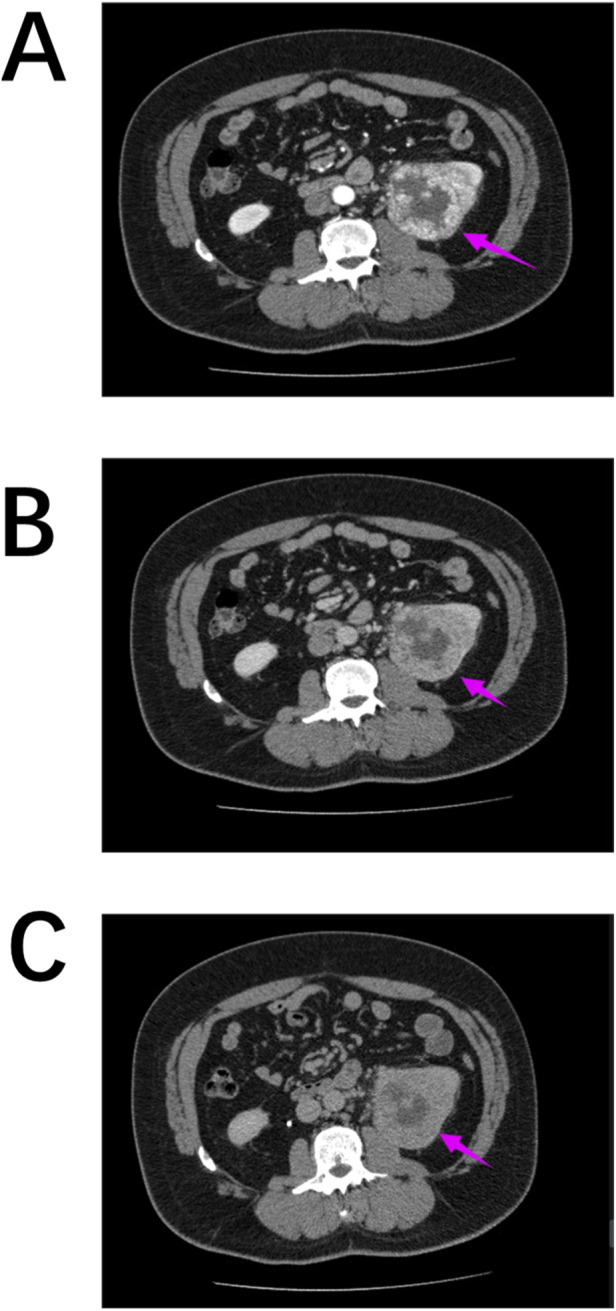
(A–C) The distinctive “wash-in, wash-out” pattern of renal clear cell carcinoma on CT scans: (A) Corticomedullary Phase; (B) Nephrographic Phase; (C) Excretory Phase.
